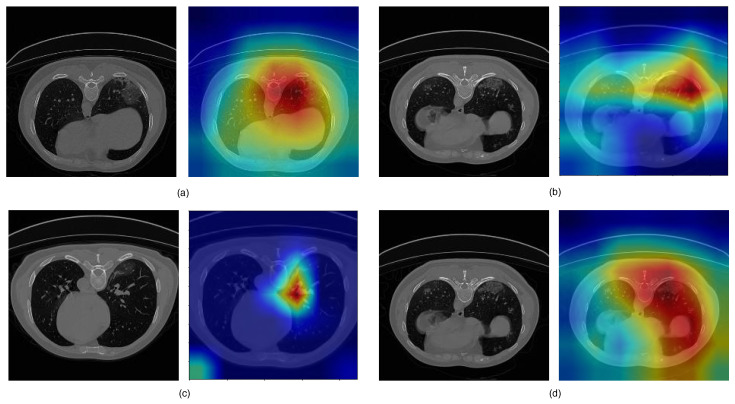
Different examples of channel attention maps (CAMs) obtained from the LungINFseg. (a–d) present examples of COVID-19 infection in CT images (left) and the corresponding CAMs (right).
